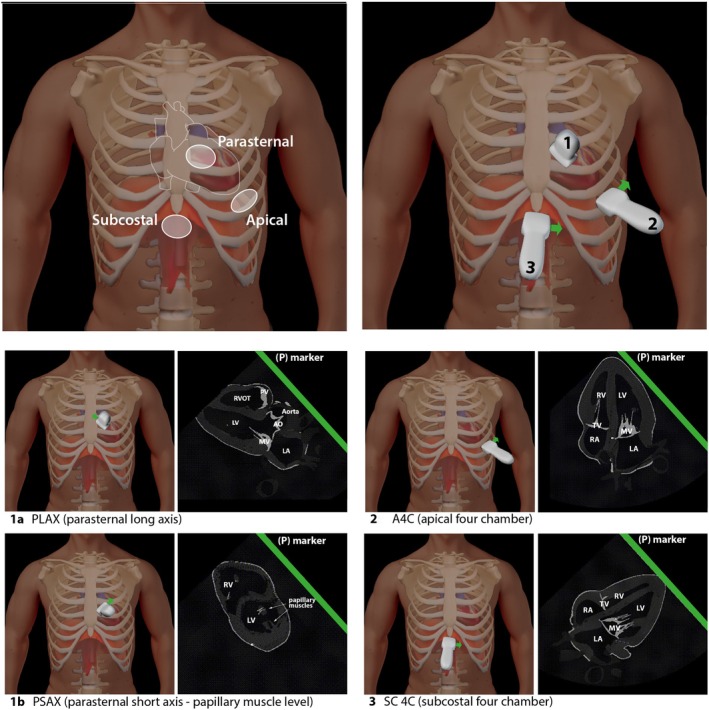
The figure displays the correct ultrasound images. The top left and right images show the probe placements on the thorax, numbered to correspond with the ultrasound images displayed below. The views are numbered and labelled as follows: (1a) parasternal long axis (PLAX), (1b) parasternal short axis (PSAX), (2) apical four‐chamber (A4C), and (3) subcostal four‐chamber (SC4AC).
